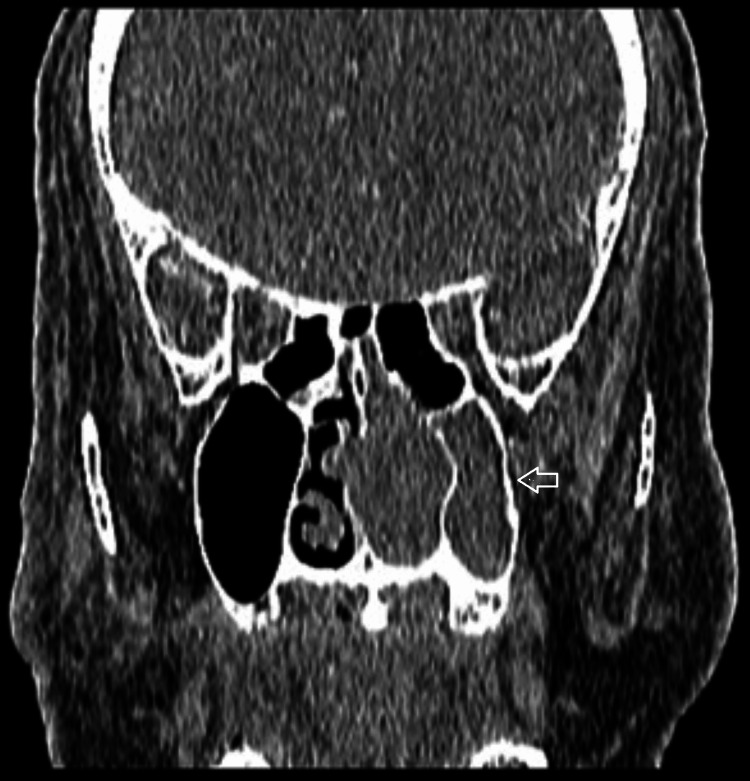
CT (coronal view) of the paranasal sinuses showing soft tissue lesion occupying the left nasal cavity with complete opacification of the left maxillary sinus (arrow). The left ostiomeatal complex is obstructed.
